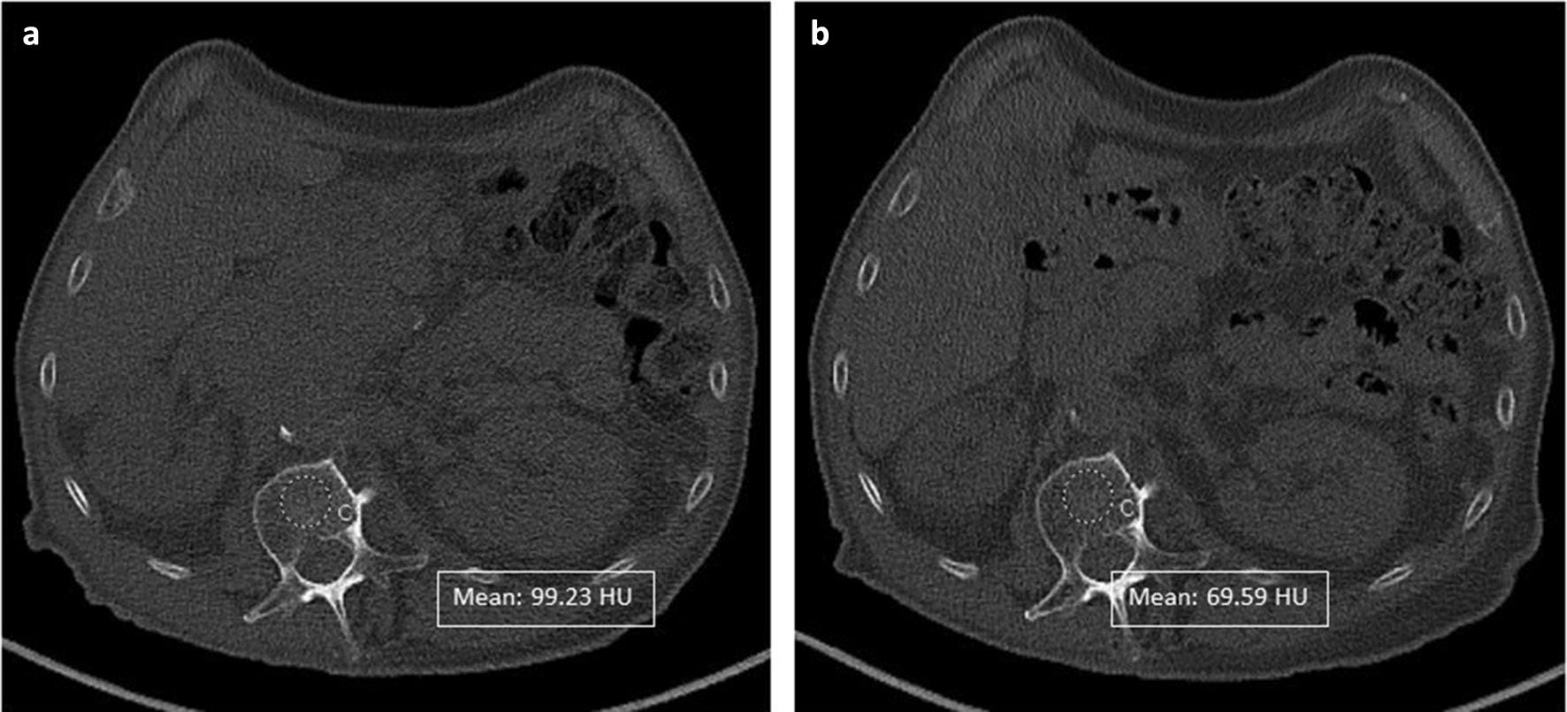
ROI placement at the level of the first lumbar vertebra (L1) in the CT images at baseline a and at the end of treatment b, showing a reduction in BMD
